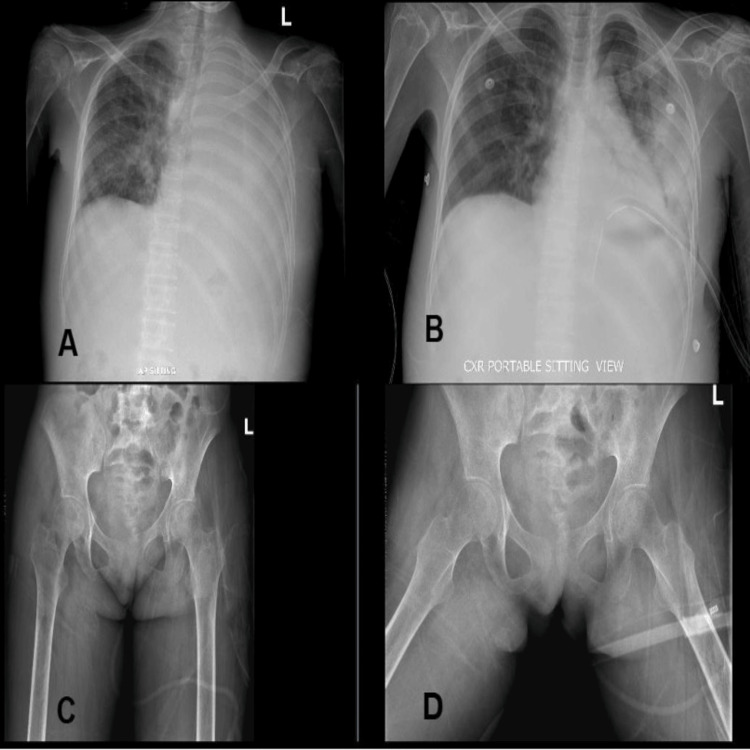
A, Left-sided pleural effusion; B, left-sided pleural effusion after chest tube insertion with reduction; C and D, bilateral hip x-ray showing diffuse osteopenia, no obvious hip joint changes
